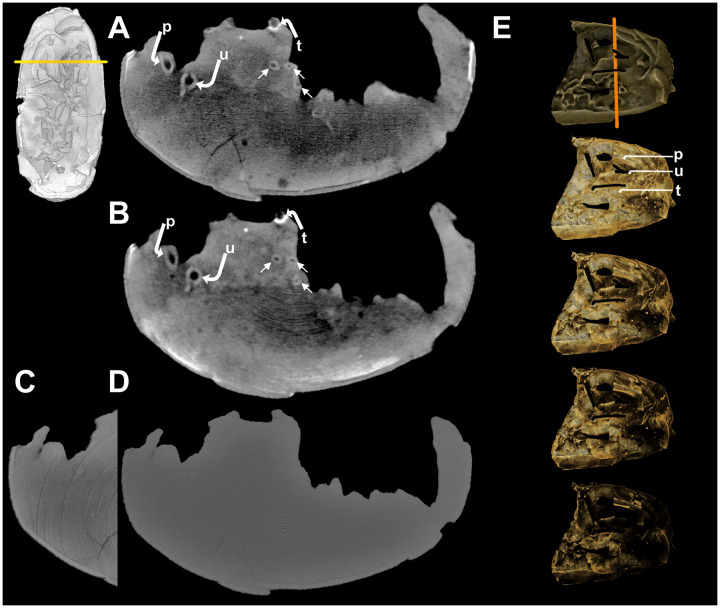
A comparison of the different tomographic imaging techniques used in this study using Egg III (SMNH-20140105-3). A cross section along the orange line of Egg III from (A) ANTARES, (B) NECTAR, and (C and D) X-ray CT with different contrasts. The arrows point out the cortical rim of the embryonic long bones in Egg III. (E) Volume renderings based on NECTAR with different thresholds for the visibility of different materials and their densities. p, pubis; t, tibia; u, unknown.
